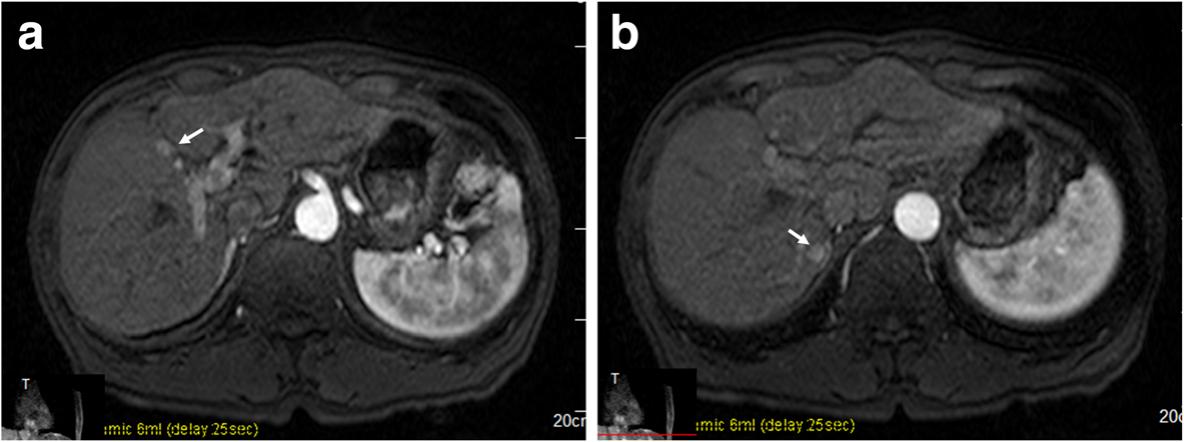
Magnetic resonance imaging shows a mass lesion enhanced during the early phase of contrast enhancement in segment 5 (a) and segment 7 (b)
